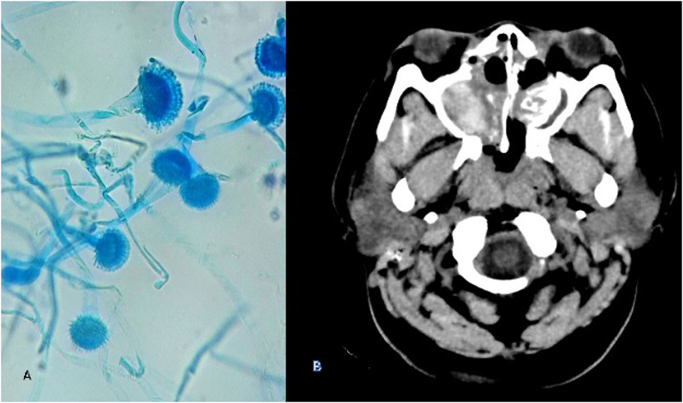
A. KOH with *Aspergillus flavus* septated hyaline hyphae. B. Brain MRI with a nasopharynx-dependent mass invasion of the anterior cerebral fossa, orbit and maxillary and frontal sinuses.
